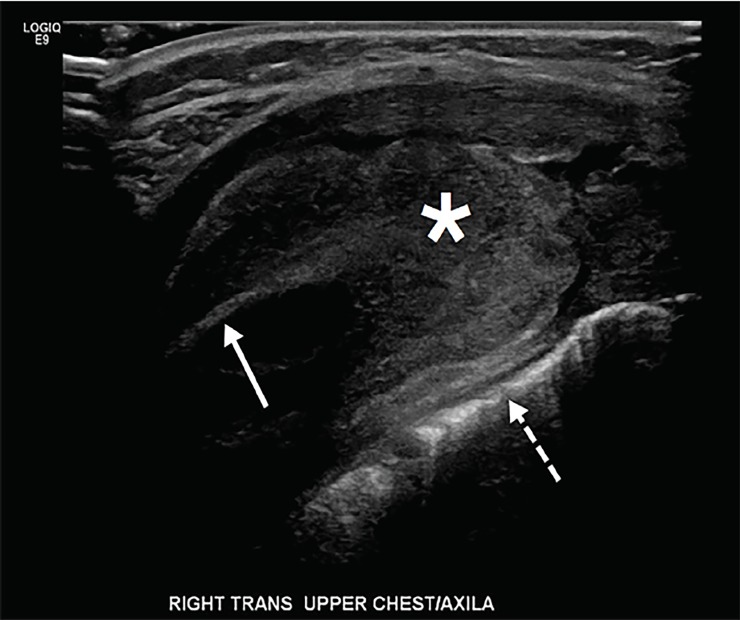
Formal ultrasound of right upper chest/axilla showing rib (arrow), lung border (dashed arrow), and periosteal abscess (*).
